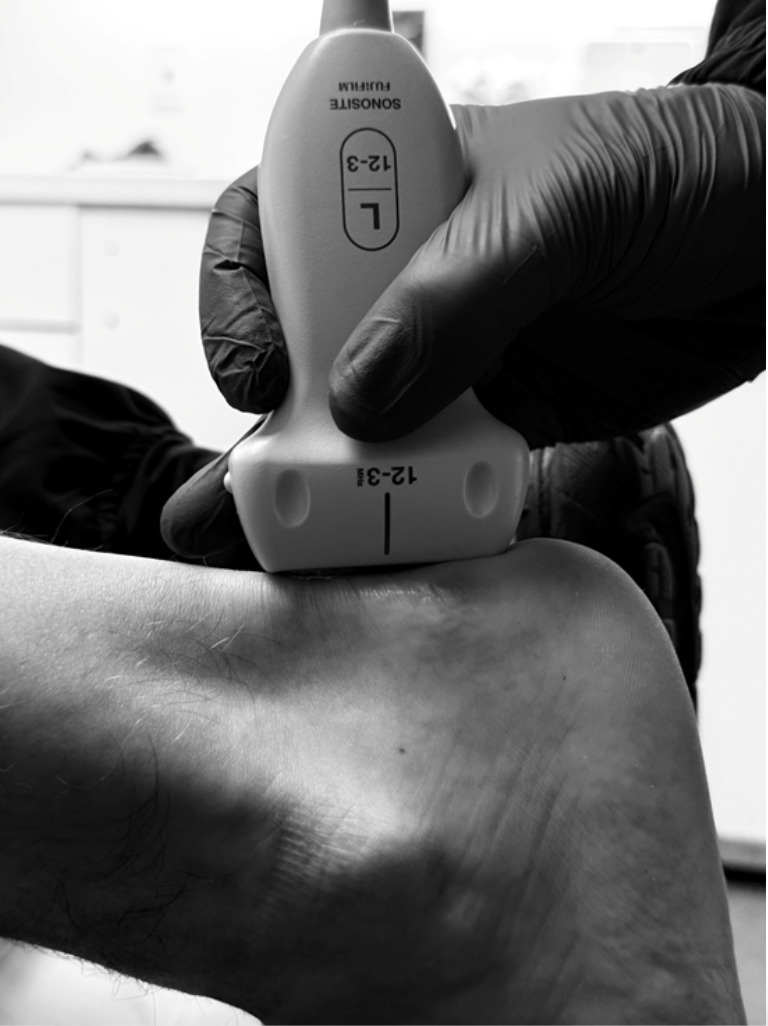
The sagittal view is obtained by placing the ultrasound probe maker toward the patient's head and scanning from the calcaneal tuberosity to the distal calf.
